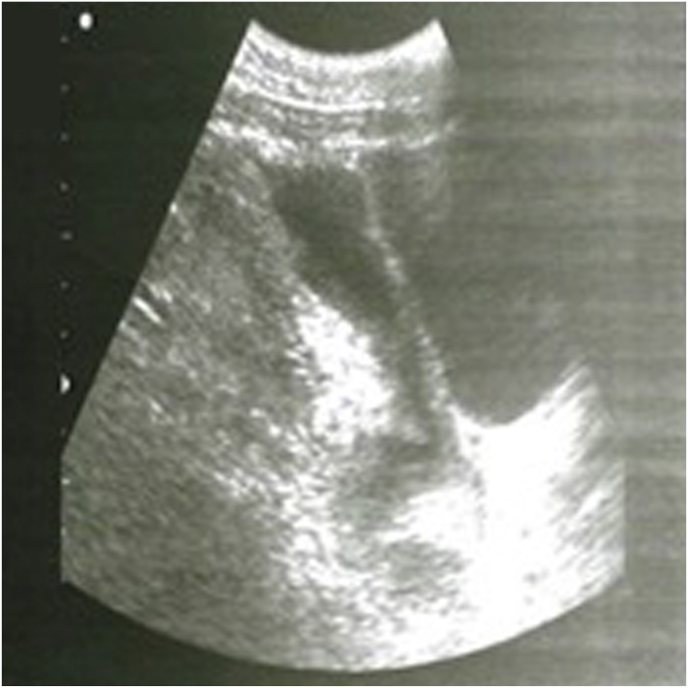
Bedside abdomen ultrasonography showing free fluids at the Pouch of Douglas.
